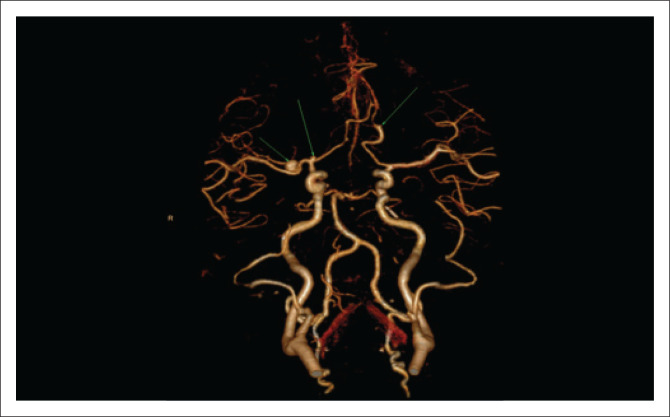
3D reconstructed maximum intensity projection (MIP) of CTA showing multiple saccular aneurysms, with the largest at the right MCA bifurcation and two smaller aneurysms at the right terminal ICA and A2 segment of the left ACA (green arrows).
